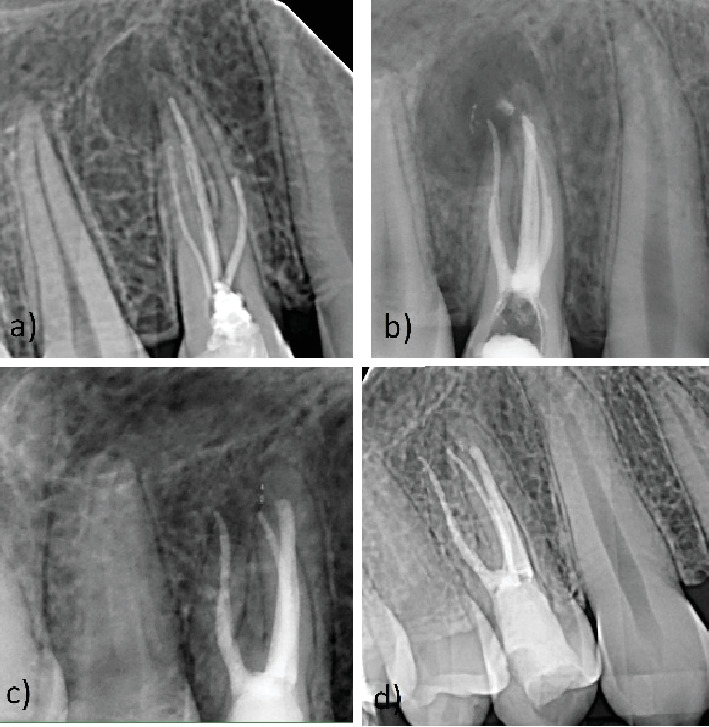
Clinical features of Case Report 2. (a) Preoperative intraoral radiograph. (b) Postoperative intraoral radiograph, a small amount of extruded CS material into the periapical tissue, note the size of the periapical lesion. (c) Twelve-month follow-up, gradual healing of the periapical lesion. (d) Twenty-four–month follow-up, complete healing of the periapical lesion.
